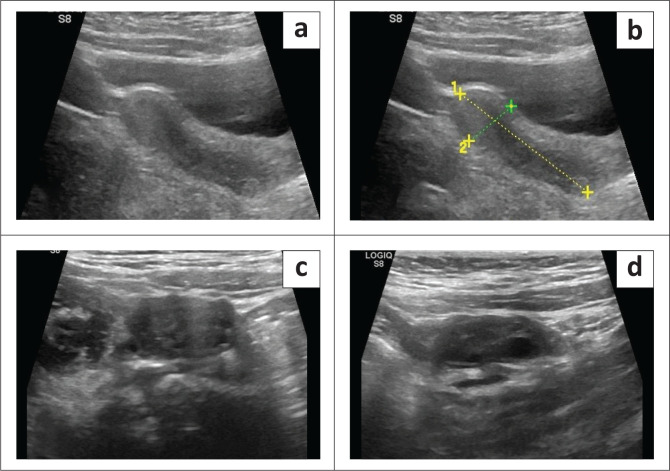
(a-d) A follow-up ultrasound after 2 months showed a significant reduction in the size of the uterus and regression in the size of both ovaries, with almost complete resolution of the cysts.
